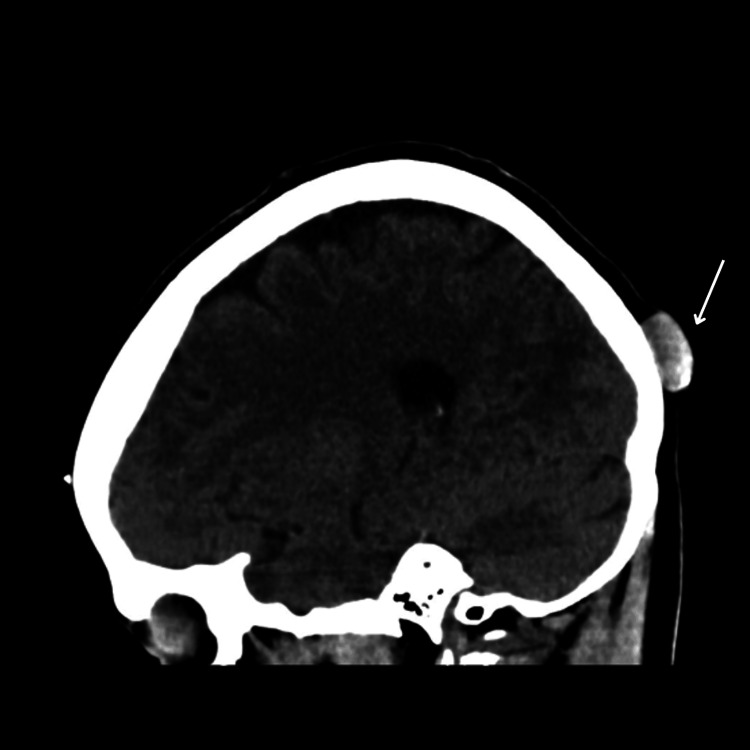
Sagittal view of the noncontrasted CT scan of head Left occipital well-demarcated round lesion with partial calcification without skull invasion (arrow) CT: computed tomography
